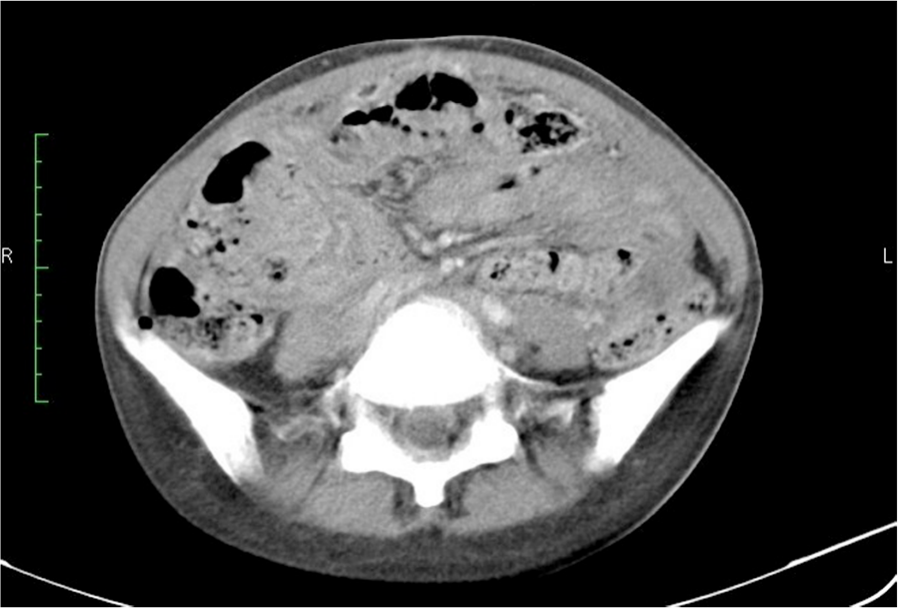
Contrast Enhanced CT of the abdomen. Matted bowel loops with mesenteric stranding and dense ascites (Hounsfield unit greater than fluid density).
